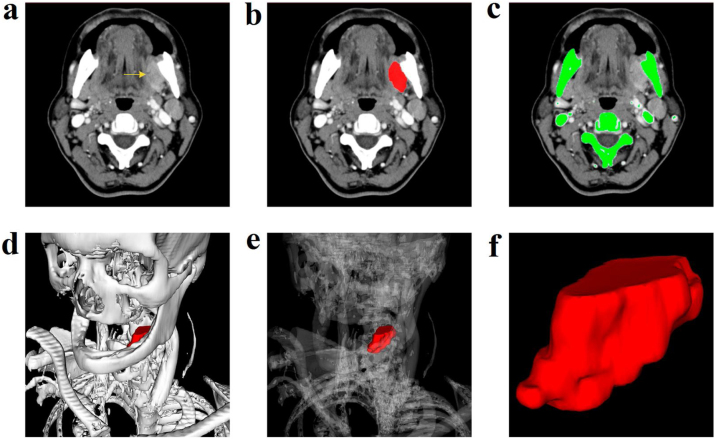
Contrast enhanced computed tomography scan: left retromolar trigone lesion (A). Manual segmentation of the tumor (B). Automatic segmentation of the skeleton (C). Tumor Three-Dimensional Segmentation (3DS) model along with modifiable bone transparency (D and E). Tumor spatial configuration (F).
